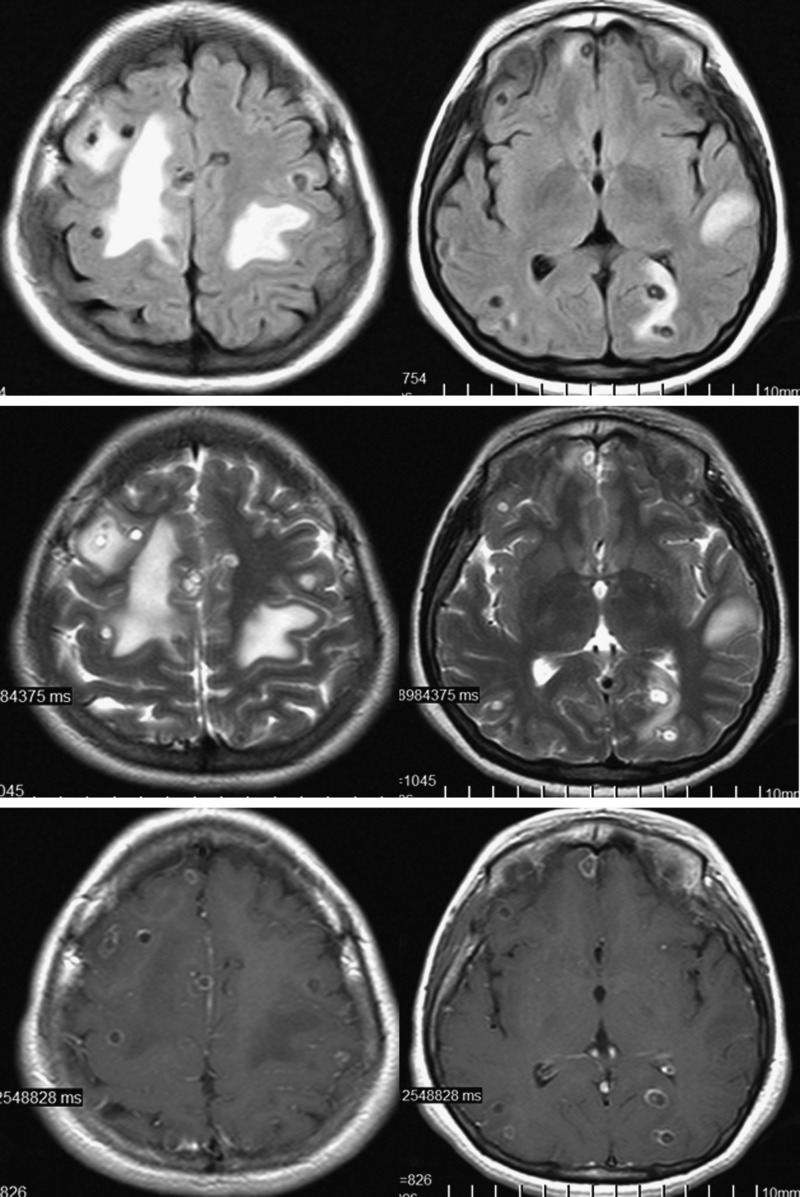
Magnetic resonance imaging of patient with encephalitic neurocysticercosis, showing multiple degenerating cysticerci and marked brain swelling.
